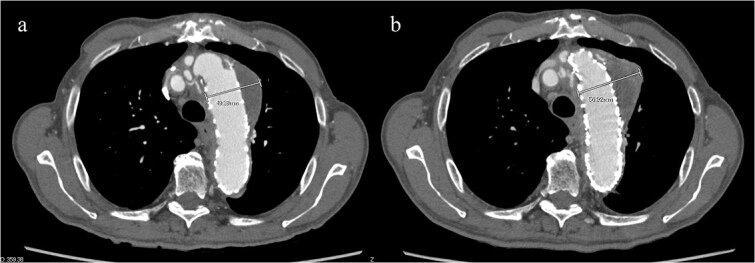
(a) Contrast-enhanced CT 1 year after total arch replacement with FET showing a reduction in aneurysm diameter from 55 mm to 49 mm, with no evidence of endoleak. (b) Contrast-enhanced CT 2 years after total arch replacement with a FET, showing an increase in aneurysm diameter to 56 mm.
